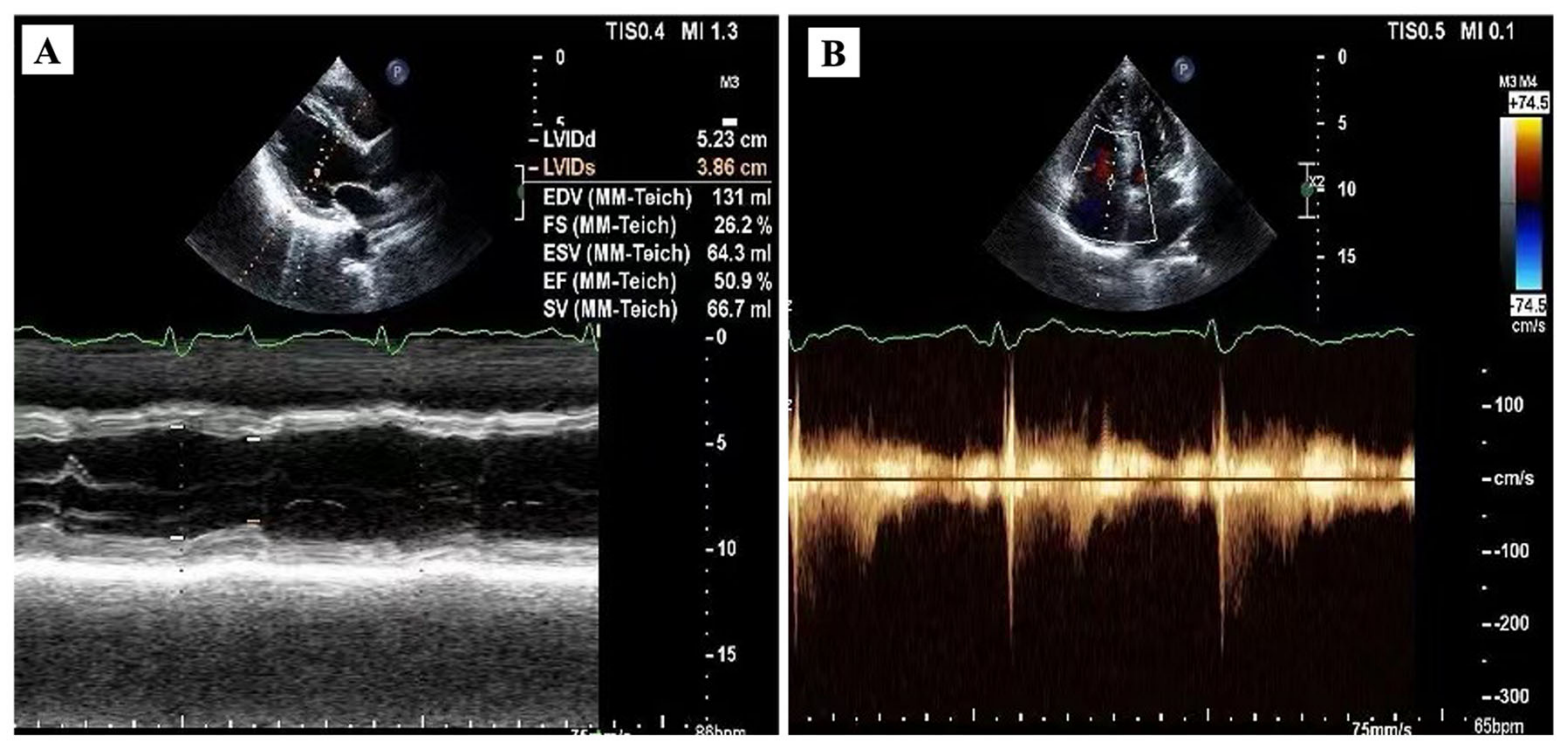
Echocardiogram during outpatient follow-up. Left ventricular ejection fraction was increased at 3 months after discharge (A). The tricuspid regurgitation was alleviated as well (B).
